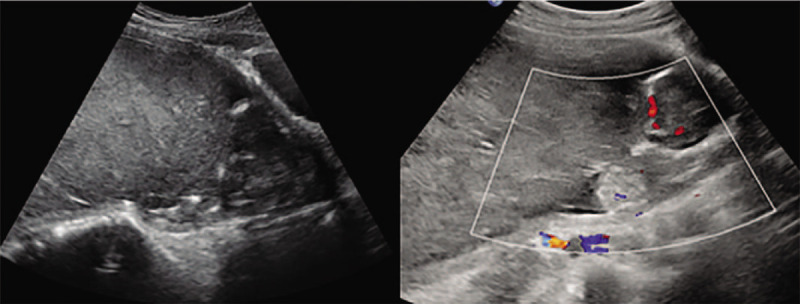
The sonogram showed a huge hyperechoic mass occupying majority of the uterine cavity and the fetus was pushed to the internal orifice of cervix.
